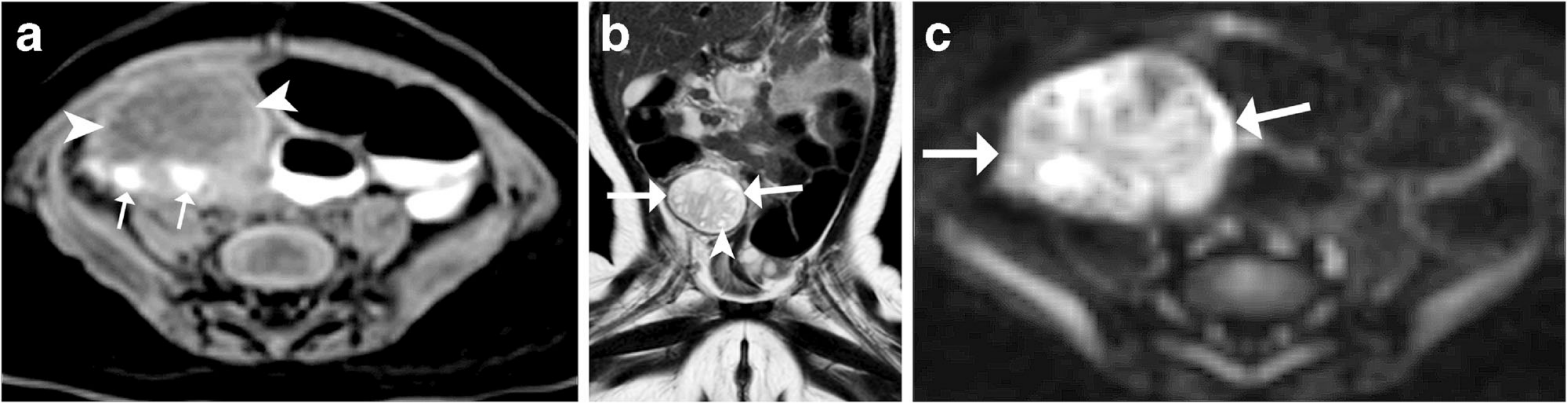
Typical appearance of ovarian torsion on MRI. in a 4 month old girl a Unenhanced axial T1-weighted fat-suppressed acquisition demonstrates hemorrhagic peripheral follicles (*arrows*) in the swollen and enlarged right ovary (*arrowheads*). b Coronal T2-weighted image shows the enlarged right-side ovary (*arrows*) with small peripheral follicles (*arrowhead*). c Axial diffusion-weighted image at b=1,000 demonstrates the diffusion impairment, inhomogenously distributed throughout the enlarged affected right ovary (*arrows*)
